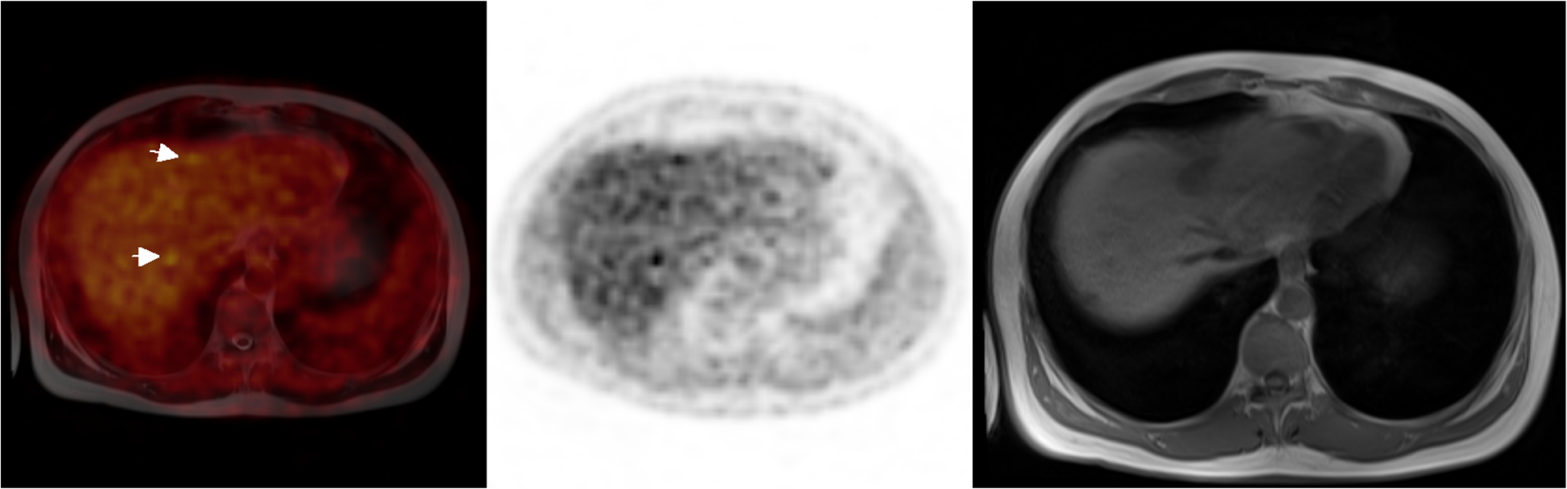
18F-FDOPA PET/MRI on the patient shows focal uptakes, suggestive of liver metastasis. The MRI/PET mismatch is depicted in this patient
